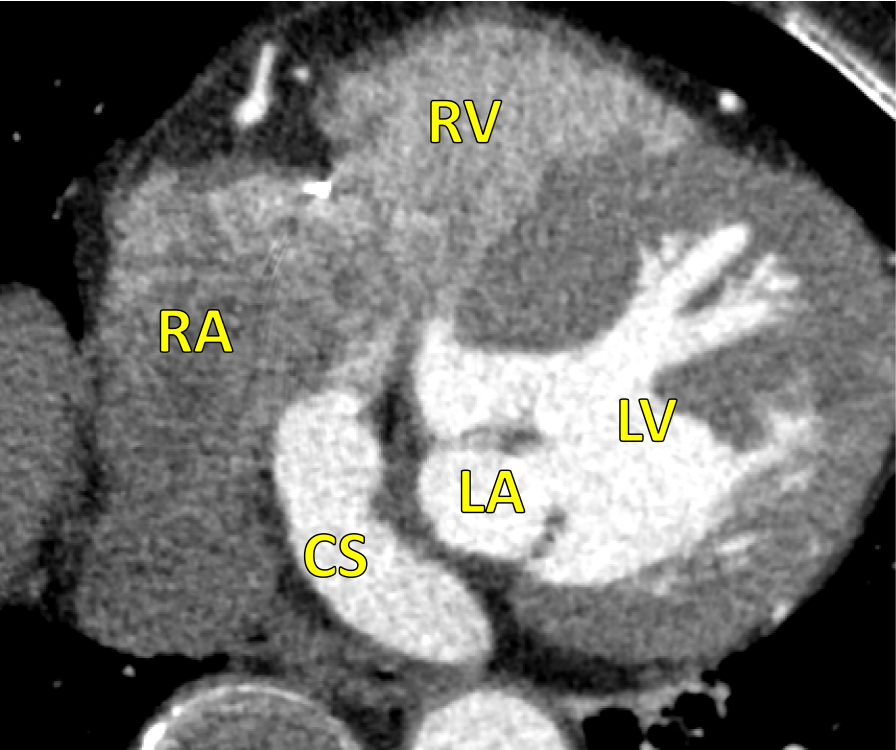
Postoperative cardiac computed tomography. Postoperative cardiac computed tomography image showing successful repair of the unroofed coronary sinus without any residual shunt. *LA* left atrium, *LV* left ventricle, *RA* right atrium, *RV* right ventricle, *CS* coronary sinus
